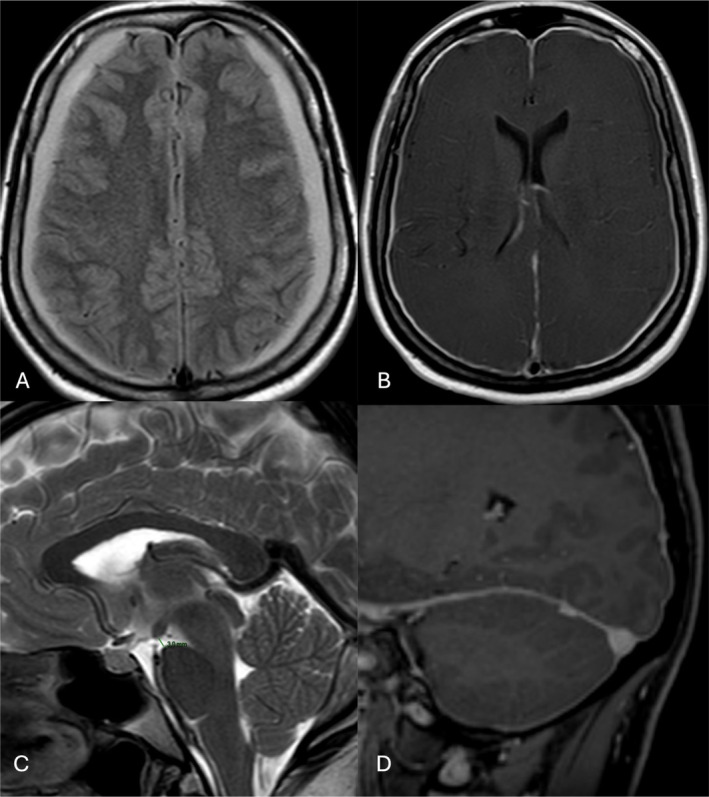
Characteristic brain magnetic resonance imaging (MRI) findings of a patient with spontaneous intracranial hypotension. (A) Axial FLAIR‐weighted image with subdural effusion. (B) Axial T1 after gadolinium injection with pachymeningeal enhancement. (C) Sagittal T2 showing sagging of brainstem with reduced mamillopontine distance (3 mm). (D) Sagittal T1 gadolinium injection with venous distension of the transverse sinus.
